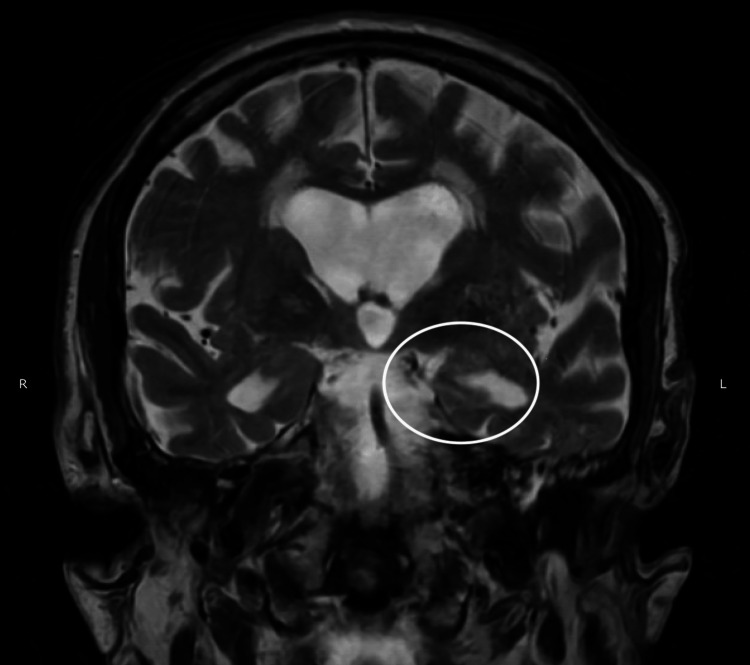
MRI brain with and without contrast coronal view The MRI (Magnetic Resonance Imaging) image is showing asymmetric prolonged T2 values at the left greater than the right hippocampal regions.
